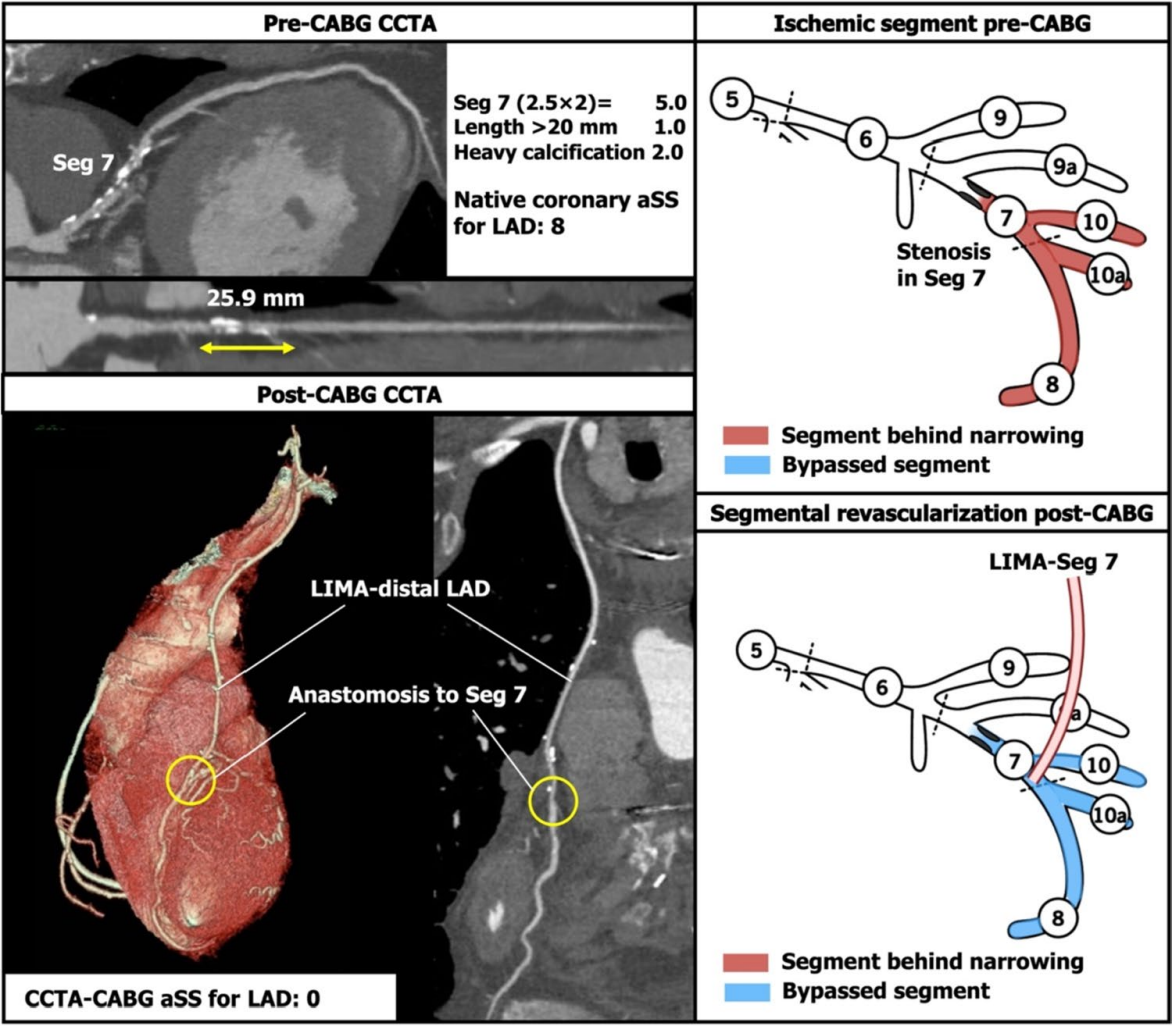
Example of points subtracted from the native coronary aSS in a left anterior descending artery (LAD) with a single lesion. If there was a lesion in segment (Seg) 7 and a bypass was placed on the distal LAD, the aSS of Seg 7 would be subtracted. *aSS* anatomical SYNTAX Score, *CABG* coronary artery bypass graft, *CCTA* coronary computed tomographic angiography, *LAD* left artery descending artery, *LIMA* left internal mammary artery
